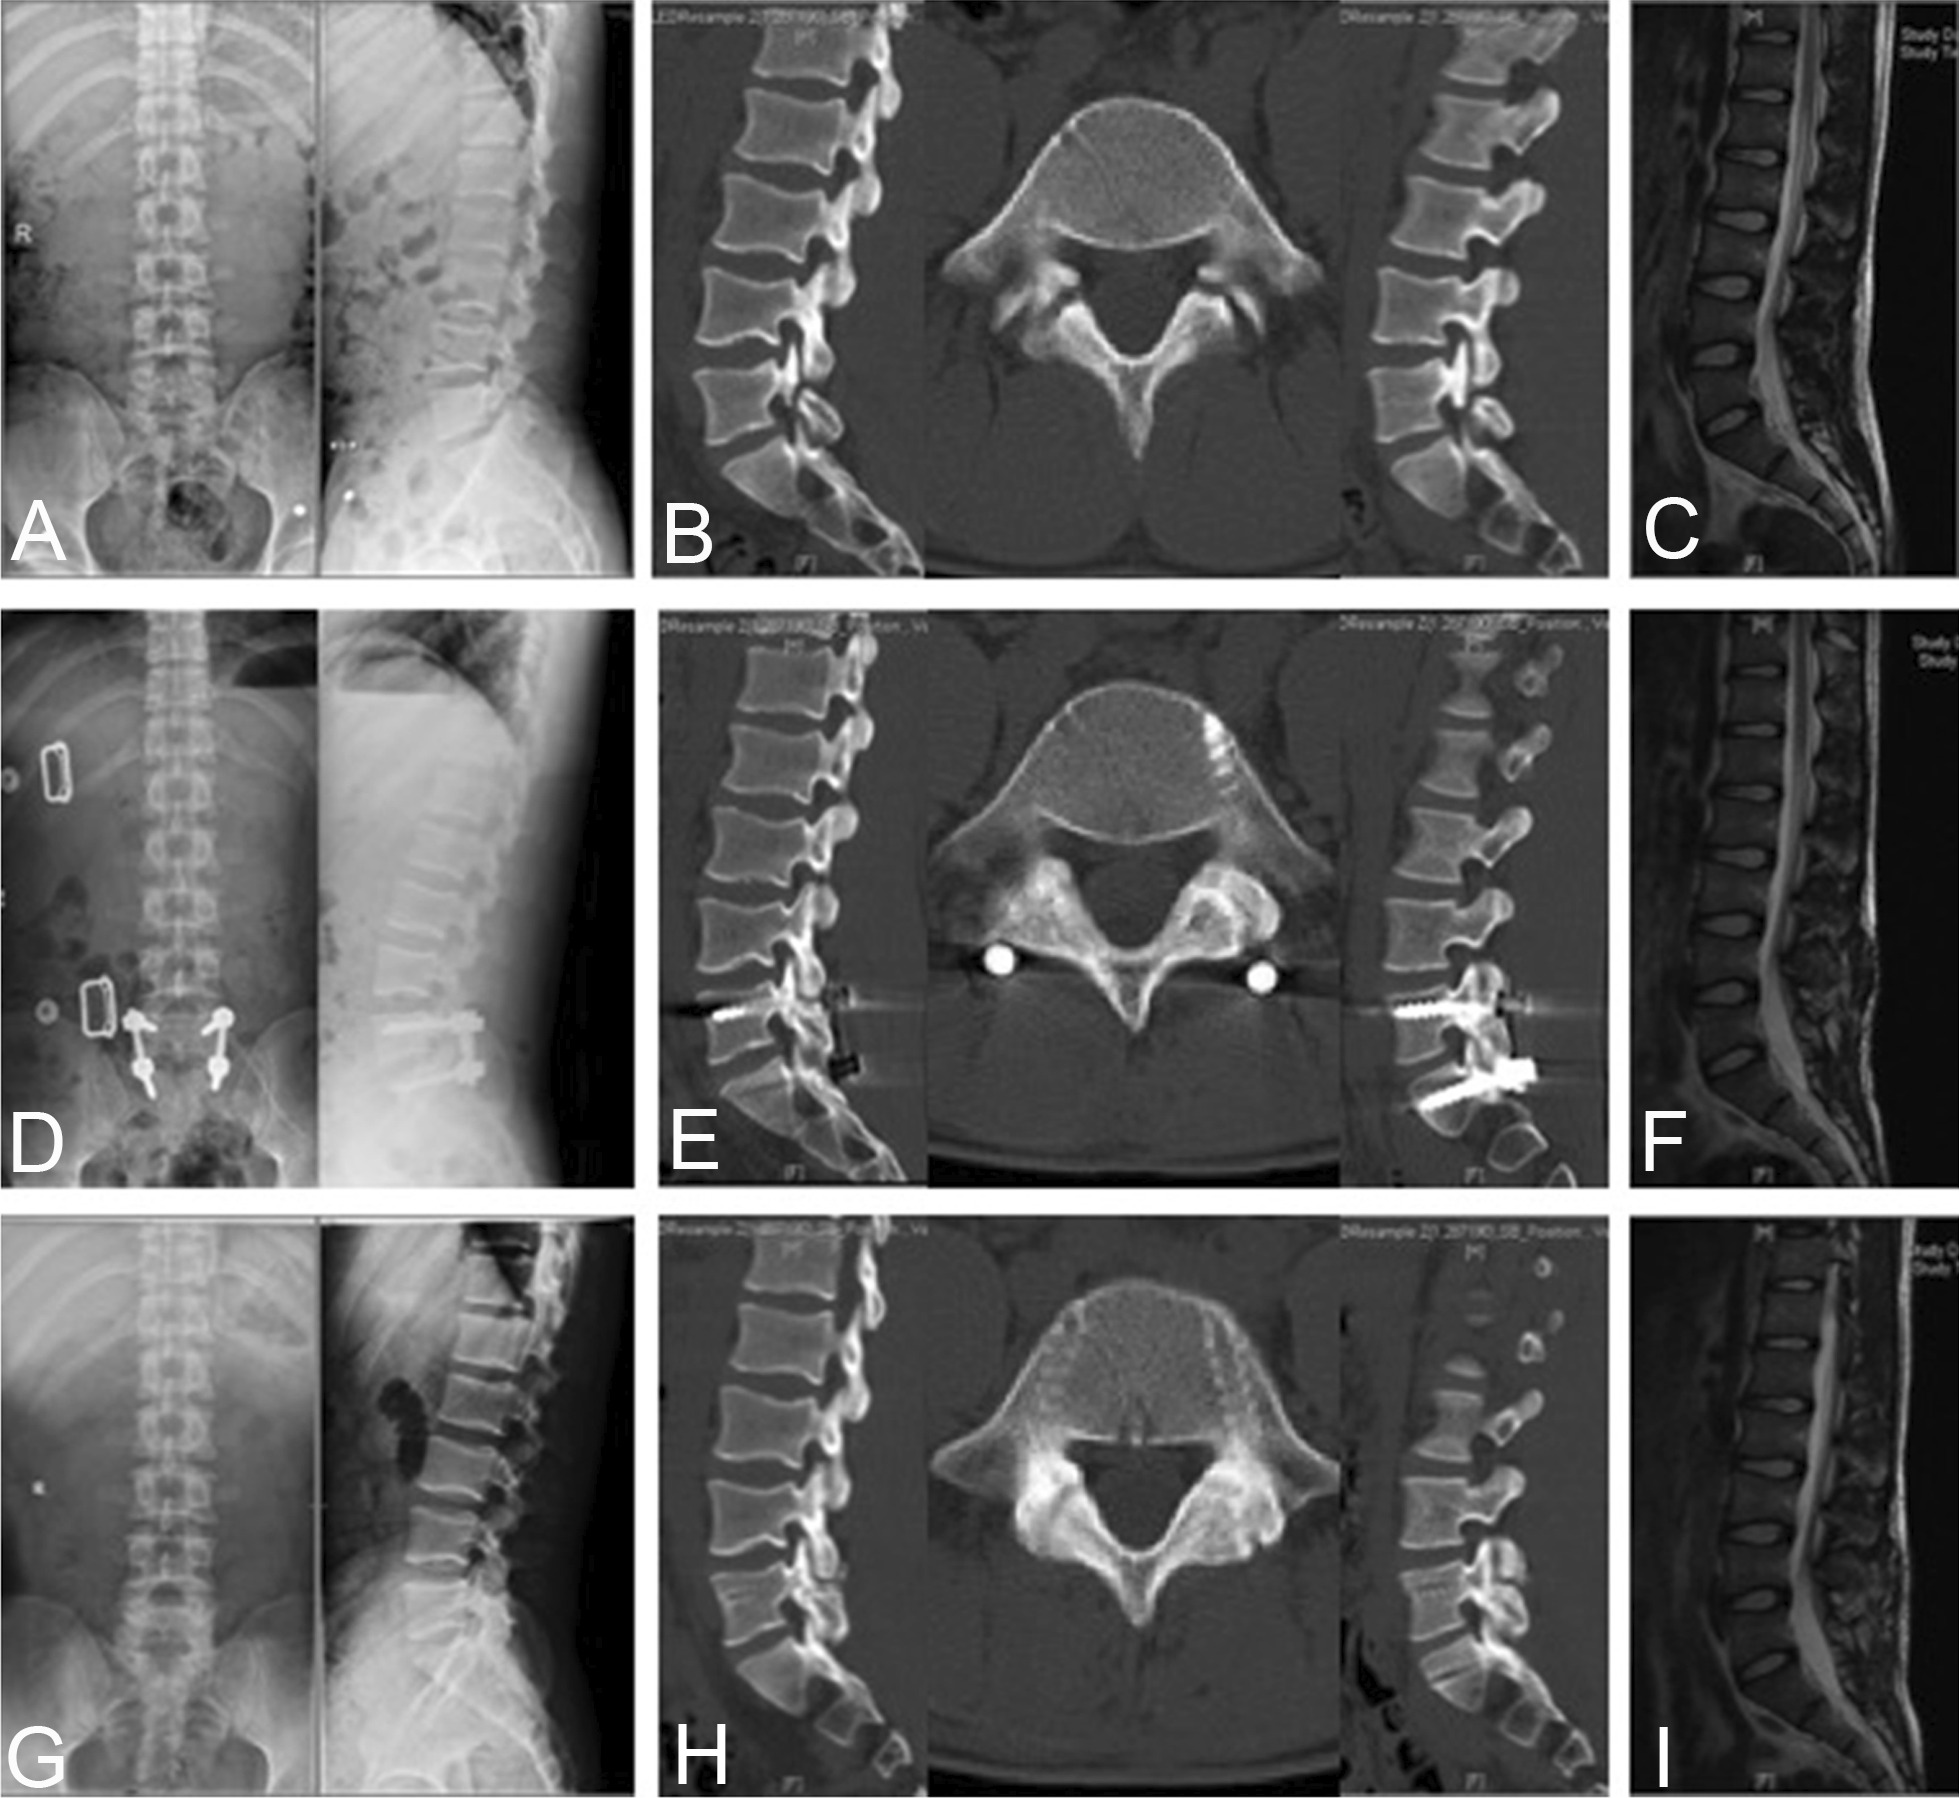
Preoperative and postoperative images obtained in a 21-year-old man with L5 spondylolysis. a–c Preoperative radiographs, CT scan and MRI. d One-week postoperative radiographs. e 12-Month follow-up CT scan showed bilateral bony union of the pars defect. f 15-Month postoperative MRI. g 15-Month postoperative radiographs showed that the lumbar instrumentations had been removed. h–i 15-Month postoperative CT scan and MRI
